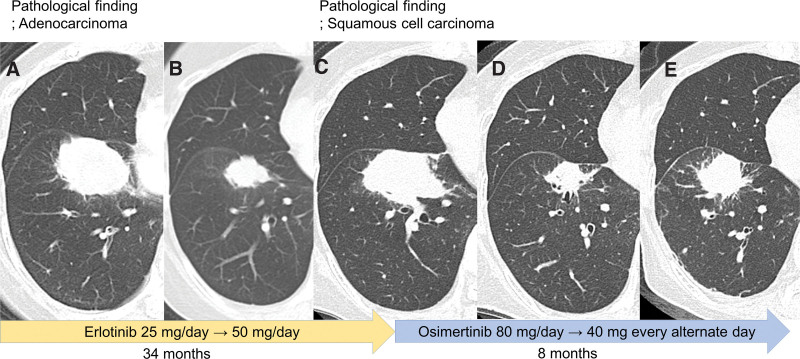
Treatment course with corresponding computed tomography (A–E). (A) before erlotinib treatment, (B) partial response after 5 months erlotinib treatment, (C) disease progression after 34 months erlotinib treatment, (D) partial response after 2 months osimertinib treatment, and (E) disease progression after 8 months of osimertinib treatment.
